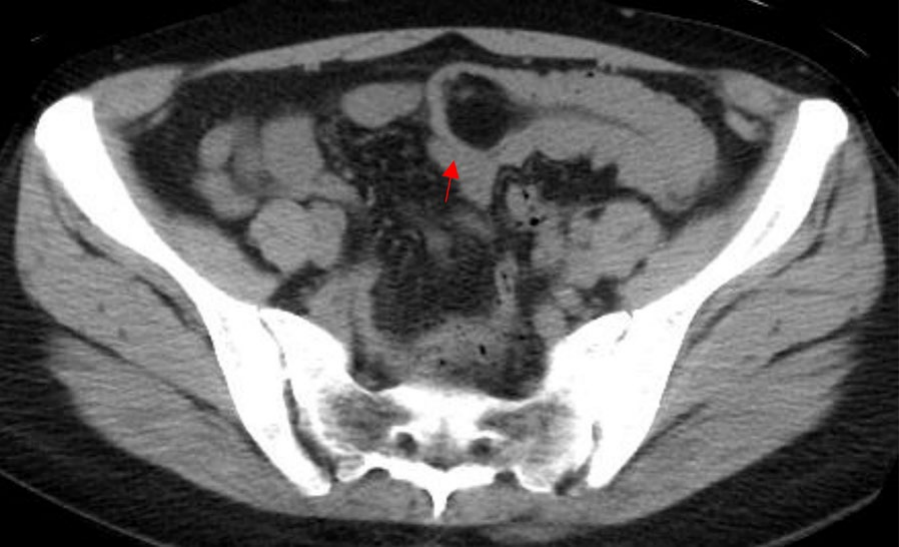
CT scans of the abdomen. A fatty mass (indicated by the red arrow), 36 mm in diameter, with a long peduncle was found in the small intestine. The pseudo-kidney sign was noted, and intussusception appeared to be present
